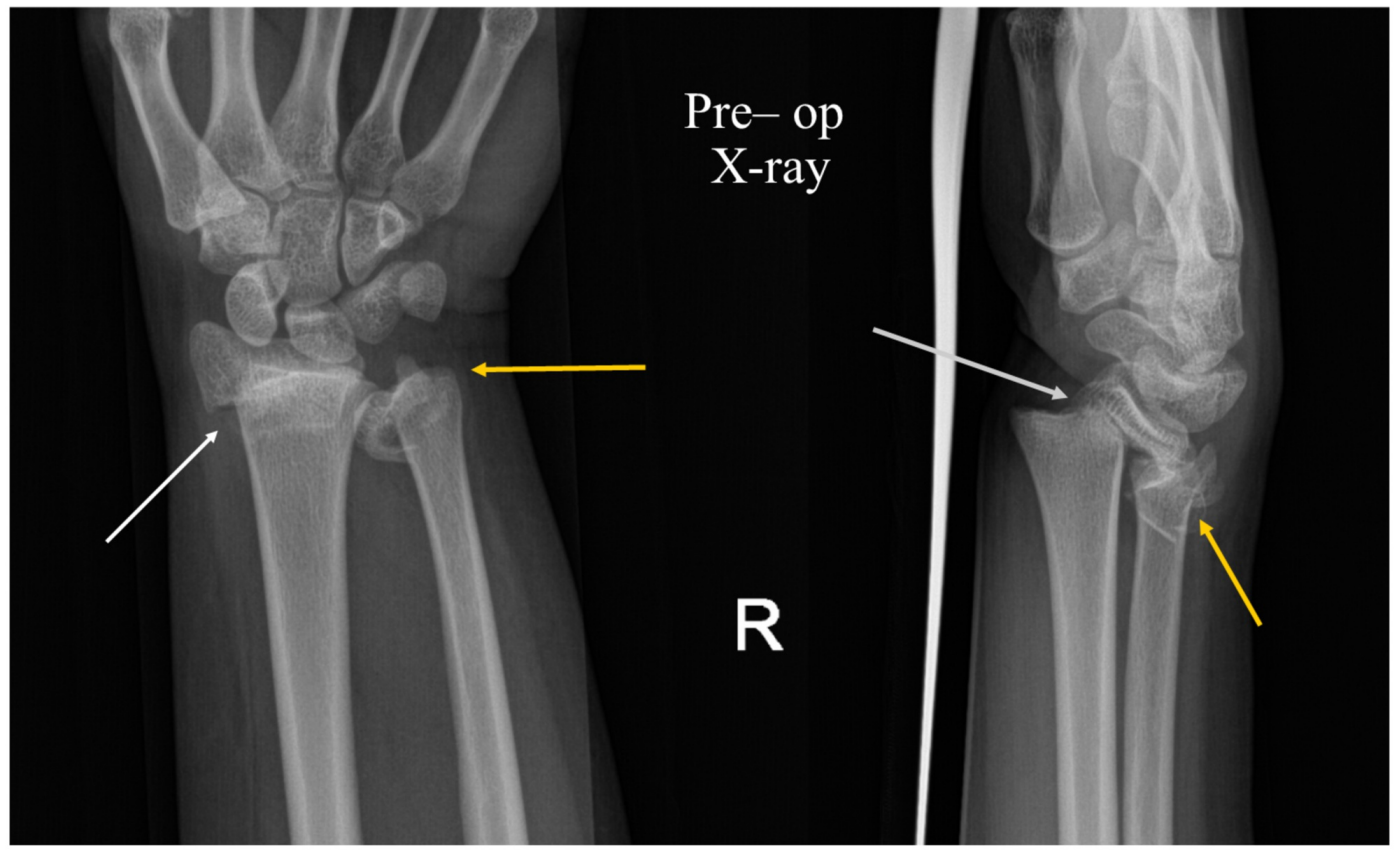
Pre-operative radiographs of the right wrist (antero-posterior and lateral views) White arrows: radial physeal injury; yellow arrows: ulnar physeal injury.
